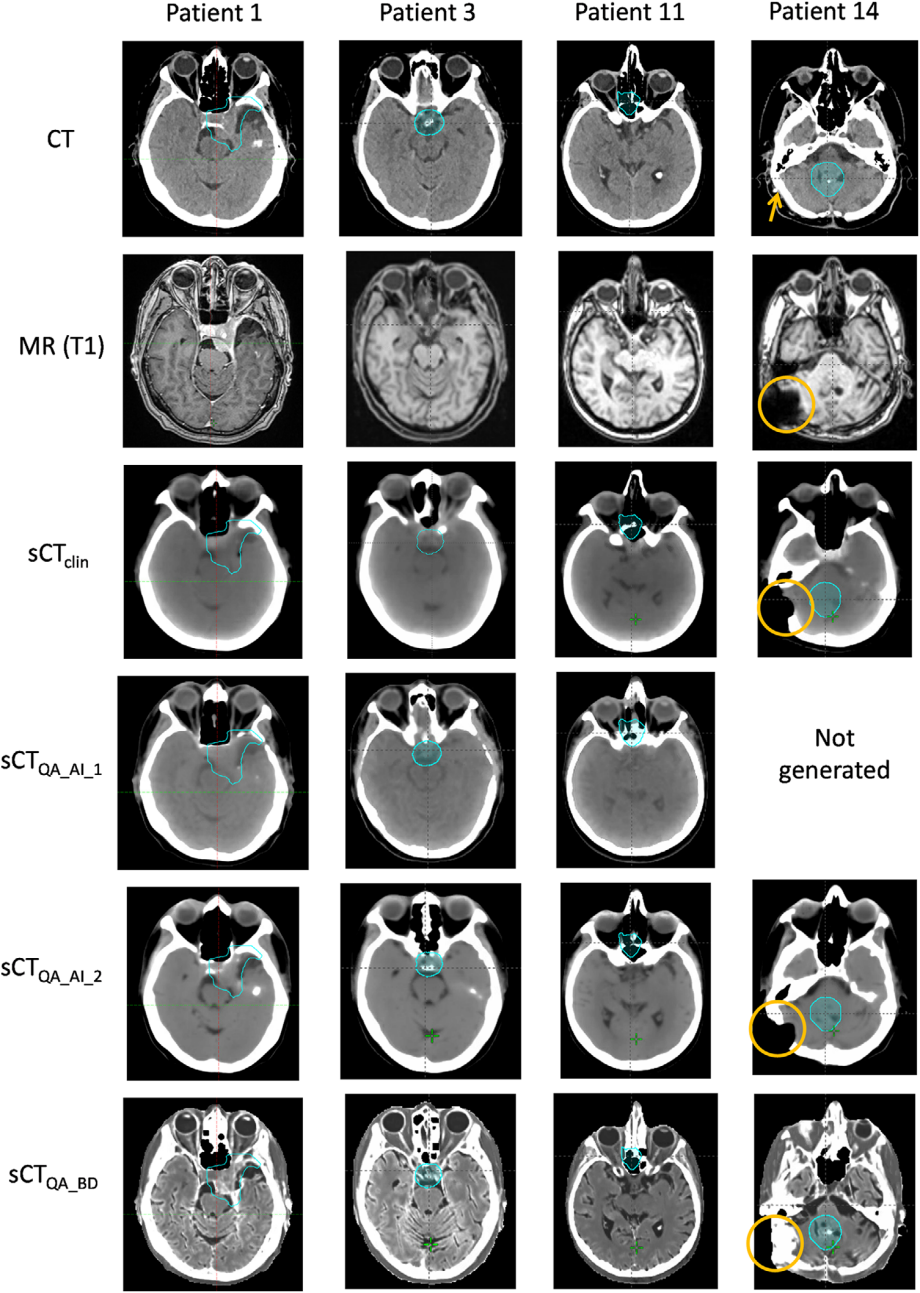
Example of axial images for the CT, MR, and sCTs studied (viewing window for CT and sCT: [− 20; 100] HU). PTV is shown in cyan. For patient 14, the metal implant and its artifact are highlighted in orange. PTV, planning target volume; sCT, synthetic CT.
